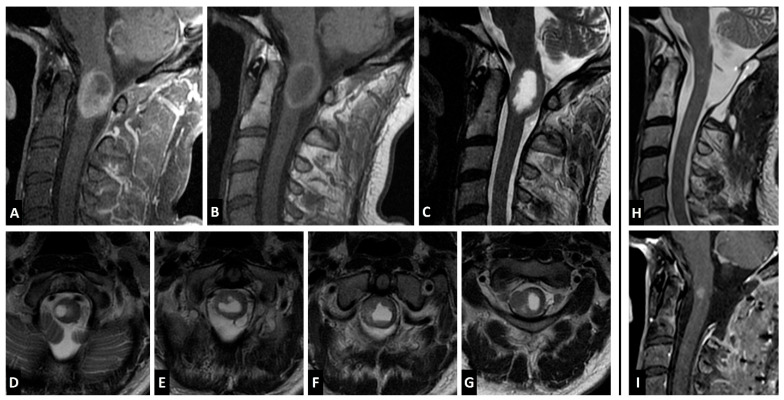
Pre- and postoperative MRI of a 44-year-old male with an intramedullary melanocytic tumor. (A) Midsagittal T1 post-Gd sequence. Note the vivid enhancement of the solid tumor component. (B) Non-Gd T1 weighted image. Note the hyperintense rim characteristic of melanin-rich pathologies. (C) T2-weighted midsagittal image, demonstrating a homogenous cystic core and the absence of cord edema or associated syrinx. (D–G) Note the lack of clear margin between the tumor and the cord parenchyma. (H,I) postoperative T2-weighted image (H) and post-Gd scan (I) demonstrating a small residual enhancing mass at the ventral aspect of the resection cavity.
